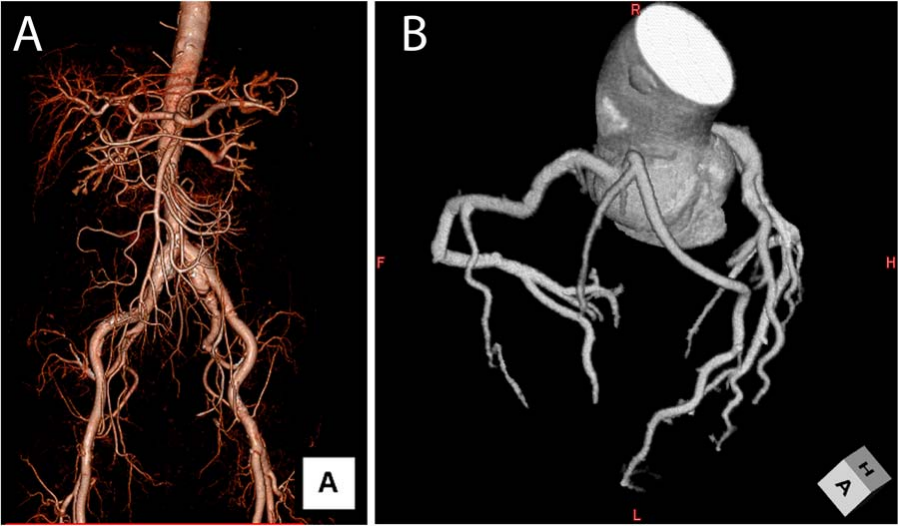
Volume rendering computed tomography. (A) Bilateral common iliac arteries were found, with a diameter of 30 mm. (B) Coronary Volume rendering CT. The anterior descending branch of the left coronary artery originated from the right coronary apex cusp, and the vessel originating from the left coronary apex cusp perfused the left diagonal and circumflex regions.
